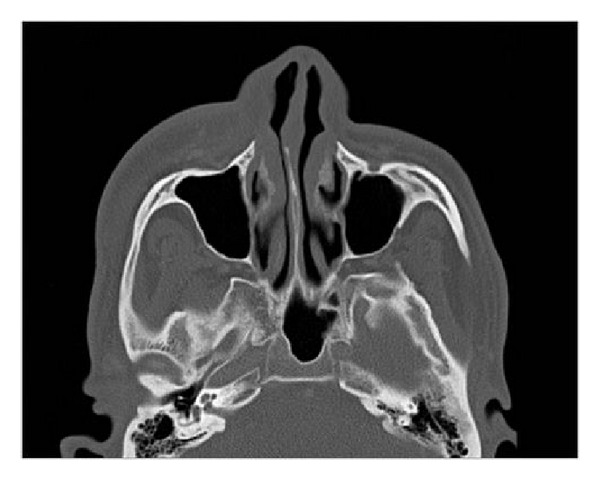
CT scan of case patient. There is soft tissue swelling overlying right maxilla. No definitive abscess is identified.
